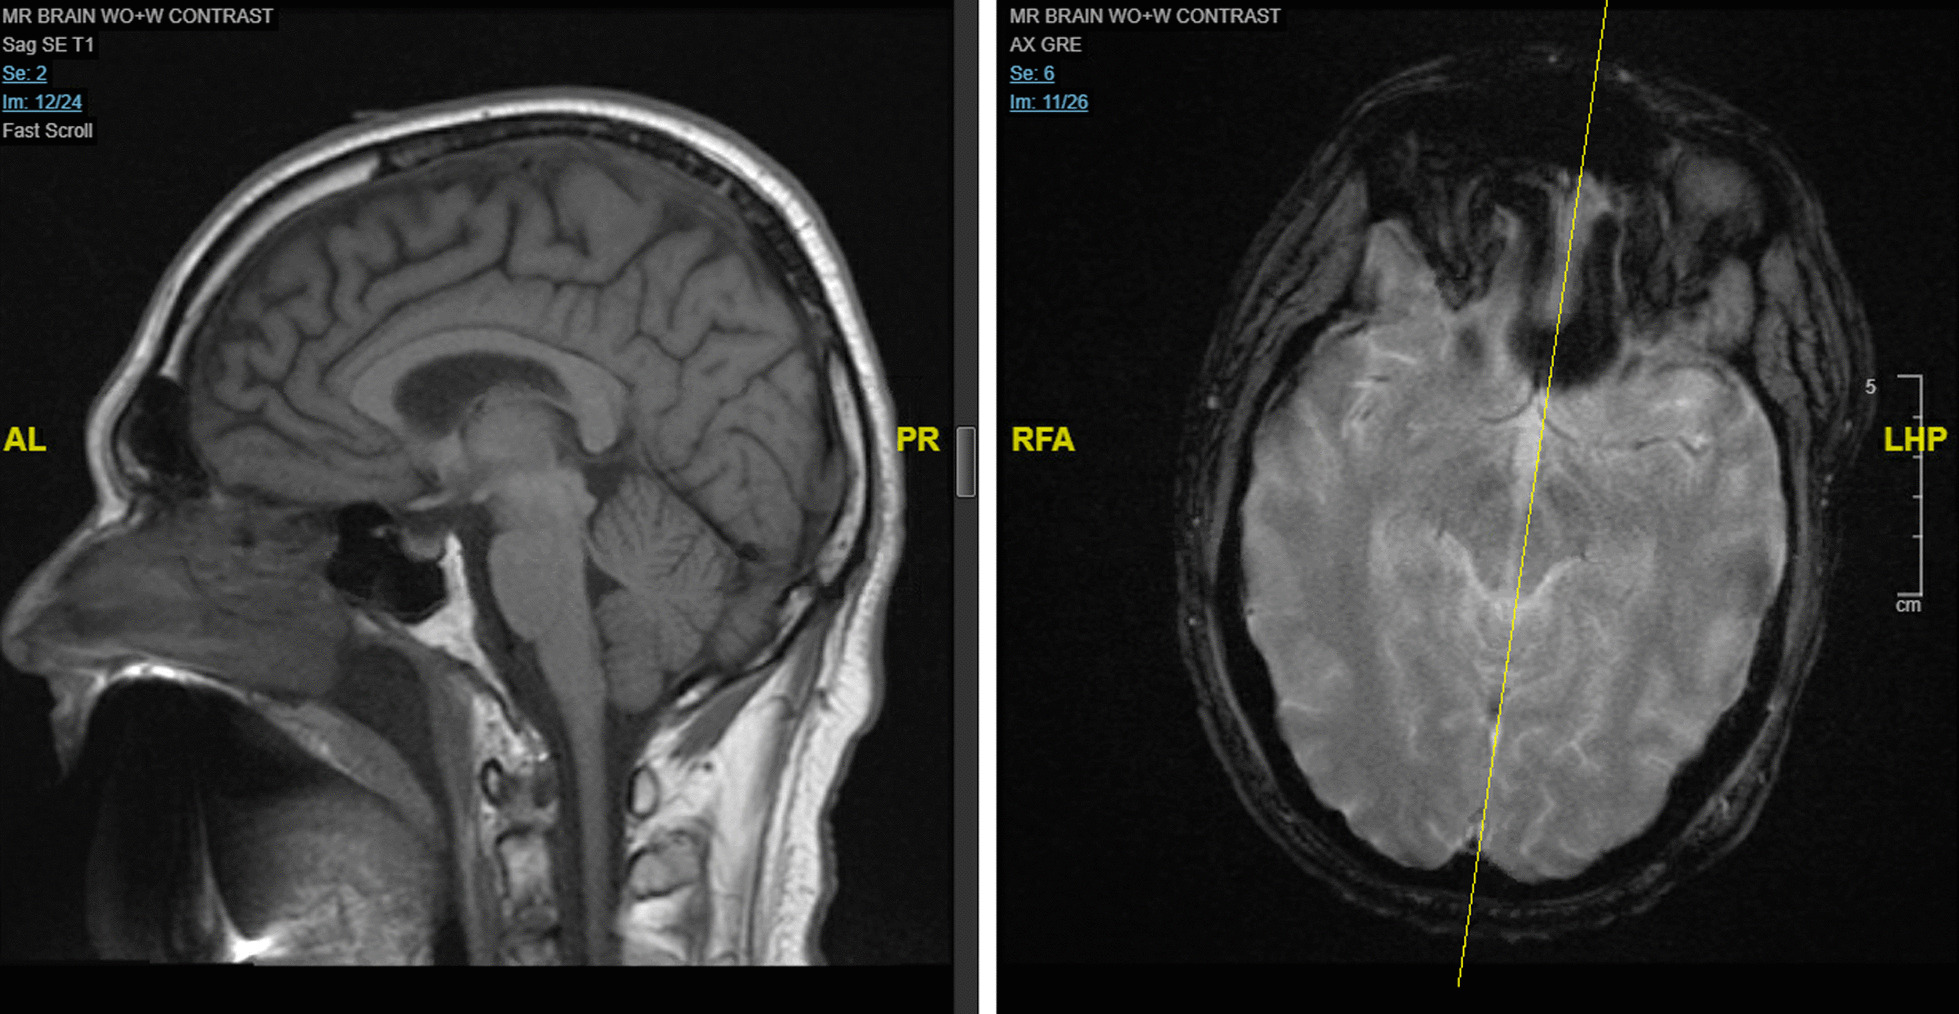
Brain magnetic resonance imaging 5 months after initial hospitalization, showing areas of signal abnormality involving the mesial temporal lobes, maxillary bodies, midbrain, and periaqueductal gray matter, which are overall similar in extent to prior brain MRI but mildly decreased in intensity
